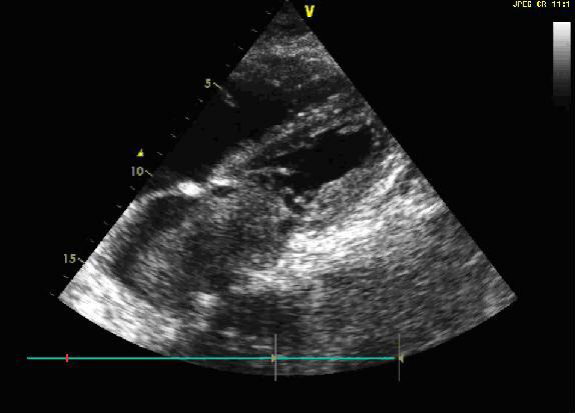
Echocardiography shows a giant left atrial mass.
